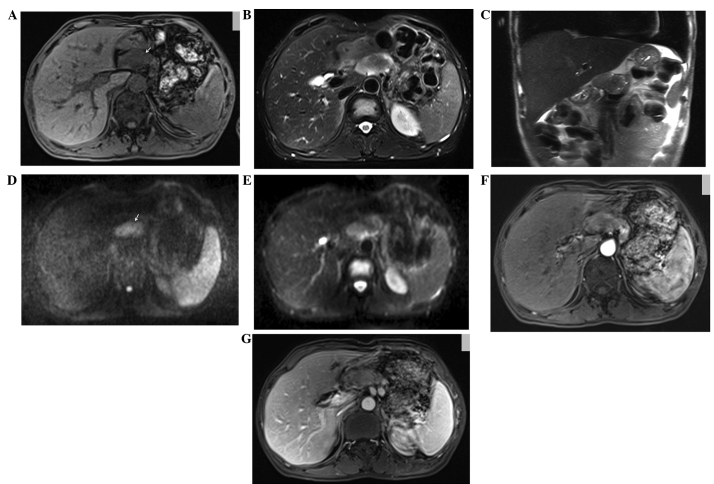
Magnetic resonance imaging manifestations of glucagonoma syndrome. The lesion exhibited a low signal intensity (arrow) on (A) T1-weighted imaging (WI), and a slightly high signal intensity on (B) T2WI (with fat-suppression) and (C) half-Fourier acquisition single-shot turbo spin echo sequence imaging. Diffusion-WI revealing the presence of a lesion with (D and E) heterogeneous hyperintensity (arrow), which was mildly reinforced during (F) the arterial phase and washed out during (G) the portal venous phase during enhanced imaging.
